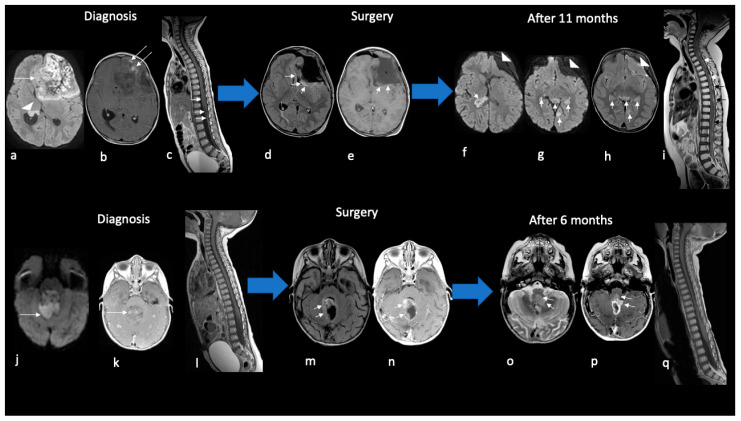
Imaging findings of disease progression in children with ATRTs. Ax DWI (a,f,g,j); Ax T1w (e); Ax T1w+mdc (b,k,n,p); Sag T1+mdc (c,i,l,q); Ax FLAIR (d,h,m); Ax T2w (o). An about 2-year-old child with frontal ATRT (a–i). At diagnosis, axial DWI MR shows reduced diffusivity of ATRT at the primary site in the frontal lobe (arrow in (a)) and at metastases in the lateral ventricle (arrowhead in (a)); mild enhancement is present (arrows in (b)). Sagittal T1+mdc MR of the spinal column shows leptomeningeal enhancement along the spinal cord (arrows in (c)). Gross total resection was performed after 10 days showing a large surgical cavity with parenchymal reactive alterations but without apparent local disease residue (arrows in (d,e)). After 7 months from surgery, the surgical cavity is collapsed (arrowheads in (f–h)); note the increase of both intracranial metastases in ventricles and perimesencephalic cistern (arrows in (f–h)) and spinal dissemination with nodular leptomeningeal foci (arrows in (i)). The patient died after 20 months. An about 1-year-old child with posterior cranial fossa ATRT (j–q). At diagnosis, axial DWI MR shows reduced diffusivity of ATRT centered in the cerebellar vermis and filling the IV ventricle (arrow in (j)); mild enhancement is detected after gadolinium (arrow in (k)) but neither intracranial nor spinal metastases are noted. Gross total resection was performed after 6 days showing the surgical cavity margined by parenchymal reactive alterations (arrows in (m,n)) without local disease residue. After 4 months from surgery local recurrence appears along the left anterolateral border of the surgical cavity (arrows in (o–p)) while no spinal dissemination is detected. The patient died after 9 months.
